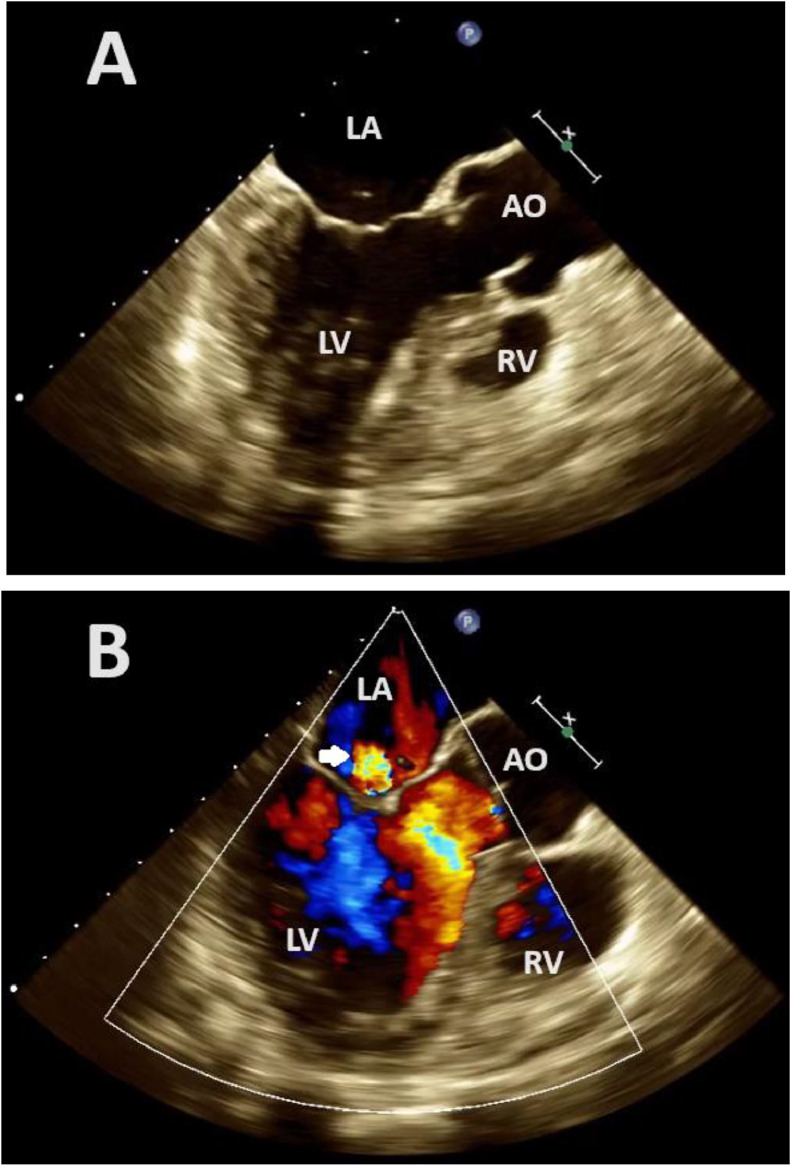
Two-dimensional transesophageal echocardiography at the mid-esophageal long-axis view during the systolic phase, without (A) and with (B) Color Flow Doppler, reveals mild mitral regurgitation (arrow). LA, the left atrium; LV, Left ventricle; RV, Right ventricle; AO, Aorta.
